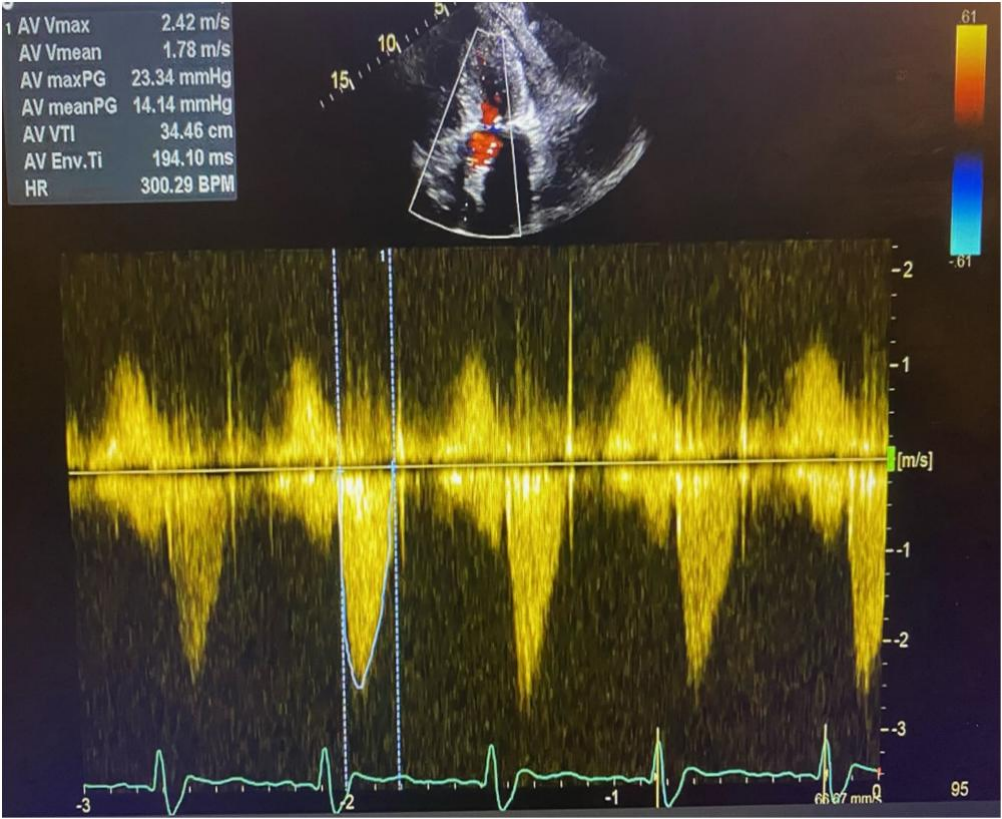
An echocardiogram post-procedure demonstrating optimal valve function.
